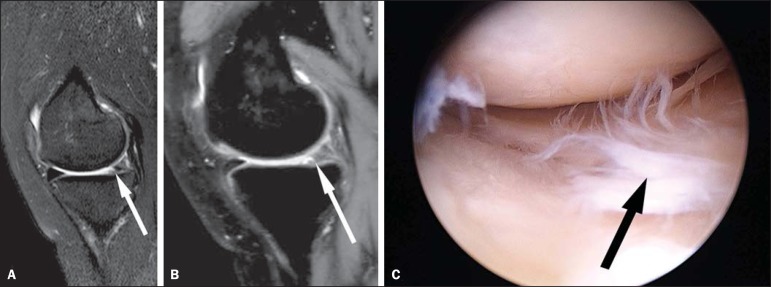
A 36-year-old woman with a tear of the medial meniscus. Sagittal MRI
